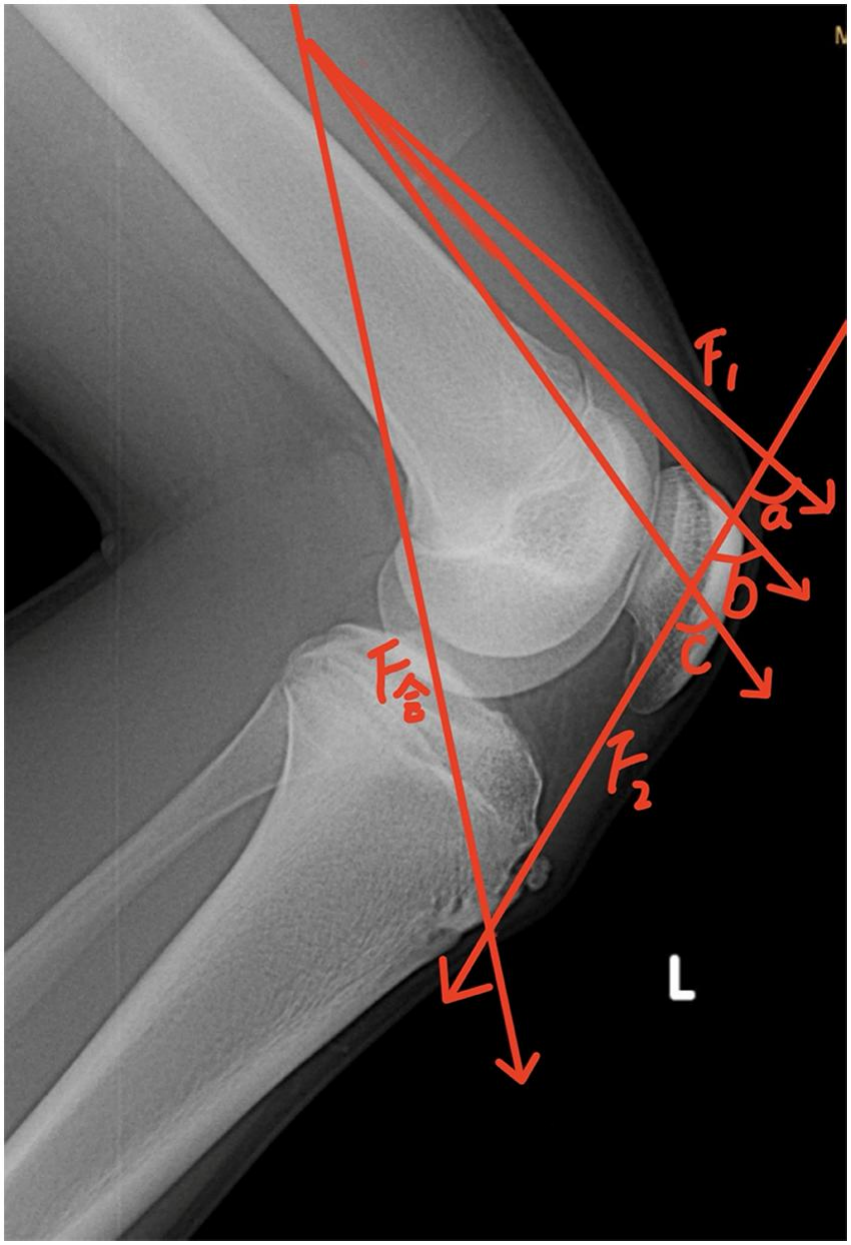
Biomechanical force vector analysis of the tibial tuberosity apophysis in OSD patients: X-ray of the flexed knee joint with labeled force components and relevant angles.
